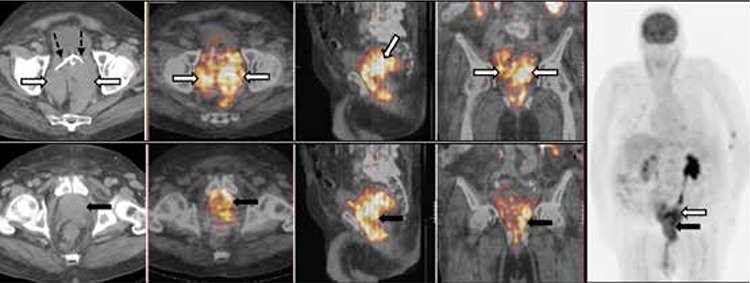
Upper row: Axial pelvic computed tomography demonstrated bilateral markedly enlarged seminal vesicles occupying most of the pelvic cavity, measuring 9x6 cm on the left and 7x4 cm on the right (white arrows), causing bilateral ureteral obstruction requiring bilateral ureteral stents (dashed arrows). Fusion fluorine-18-fluorodeoxyglucose (^18^F-FDG) positron emission tomography/computed tomography (PET/CT) (axial, sagittal and coronal) demonstrated heterogeneous intense ^18^F-FDG uptake (SUV_max_: 7.2) throughout the markedly enlarged seminal vesicles (white arrows). The patient had an indwelling urinary catheter, hence no significant retained urinary tracer activity was seen in the bladder and no obvious abnormal tracer activity was seen in the bladder wall, Lower row: Axial pelvic CT demonstrated mildly enlarged prostate gland. Fusion ^18^F-FDG PET/CT (axial, sagittal and coronal) demonstrated diffusely increased ^18^F-FDG uptake in the prostate gland with direct invasion of the pelvic floor not excluded (black arrows), Anterior whole body MIPs image demonstrated no suspicious focal FDG uptake outside the pelvis to suggest a primary lesion elsewhere (including the lungs) or metastatic spread. Low-grade focal uptake in the anterior ends of the right 3^rd^-5^th^ ribs was consistent with prior trauma
